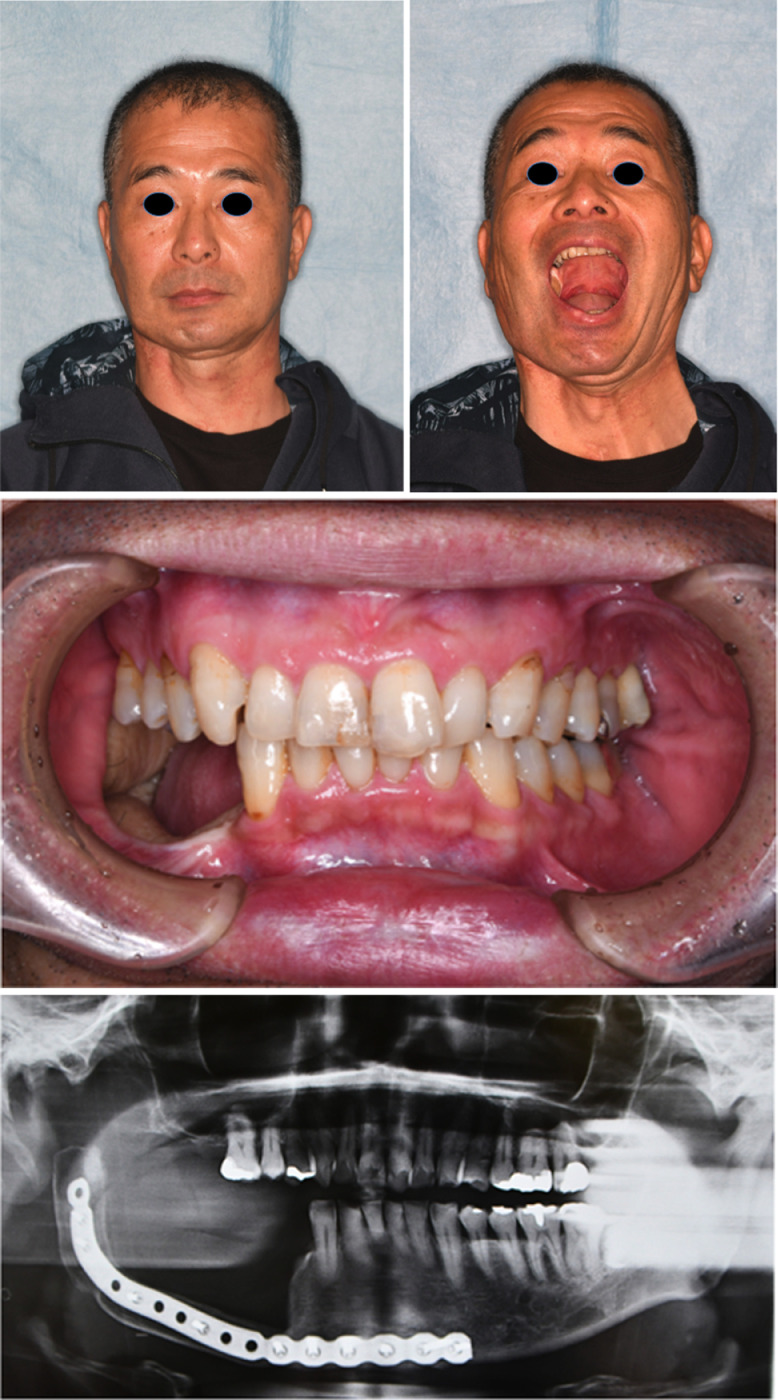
Patient 2—48 months post-surgery. (A) Extraoral postoperative photograph showing the anterior view. (B) Extraoral postoperative photograph showing the anterior view with mouth open. (C) Intraoral photograph showing good postoperative occlusion. (D) Postoperative panoramic radiograph. The distal end of fibula is seen to be located at the base of the condyle.
